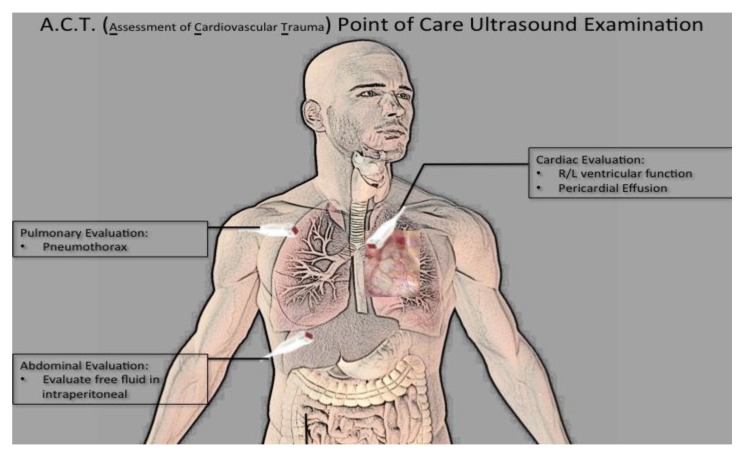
Focused ultrasound examination for assessment of cardiovascular trauma.
